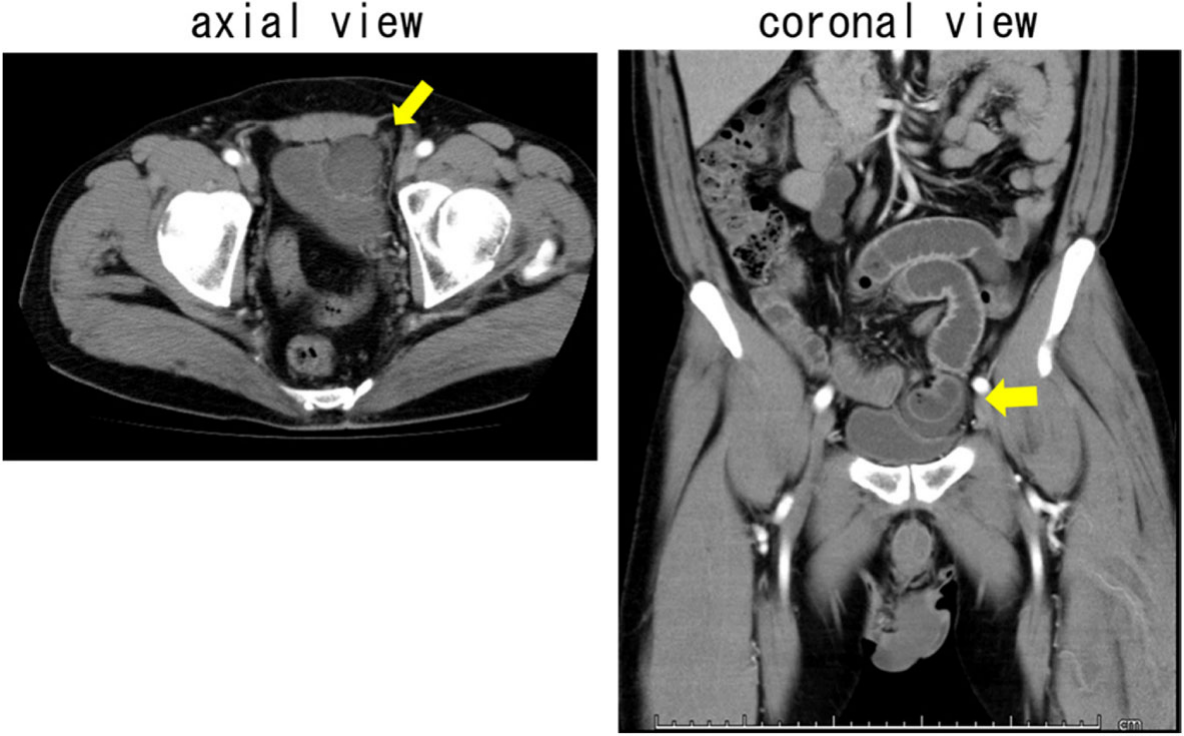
Abdominal CT findings at the time of initial examination. The left side of the bladder was depressed and dilated small intestine was found in the same part, as well as ascites fluid (arrow)
